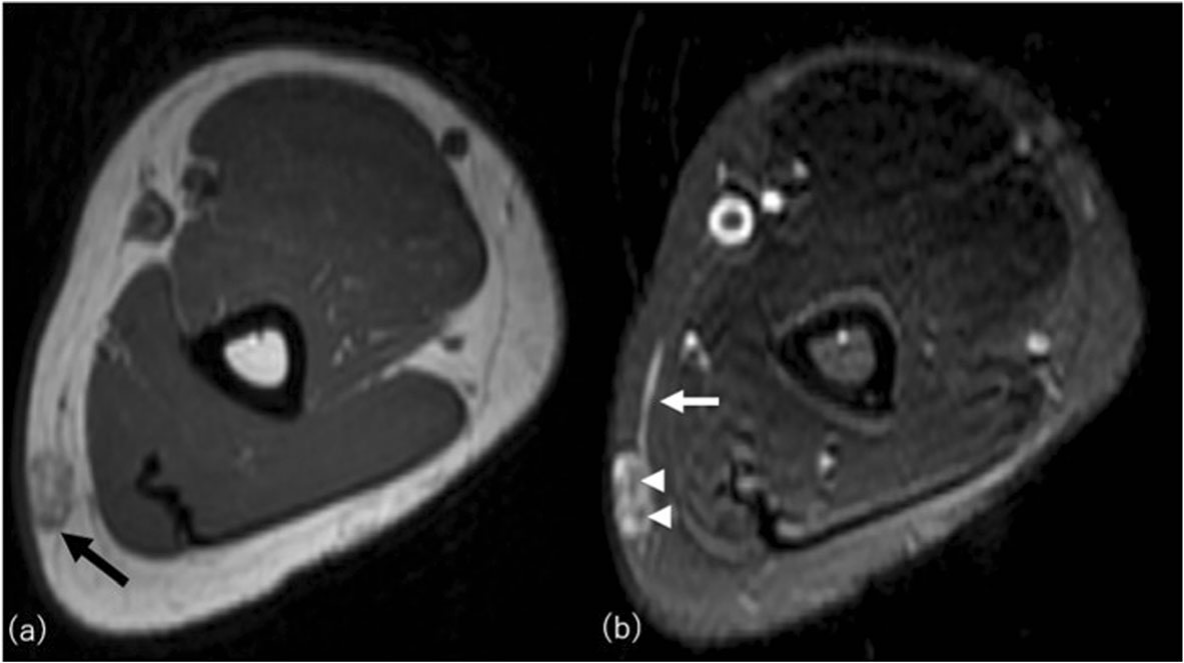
Angiolipoma in a 29-year-old man on the left upper arm. a T1-weighted image shows a subcutaneous tiny mass with inhomogeneous high to intermediate signal intensity (arrow). b Fat-saturated T2-weighted image shows hyperintense signal with focal fat suppression (arrowhead) in the mass with connection to dilated subcutaneous vein (small arrow)
